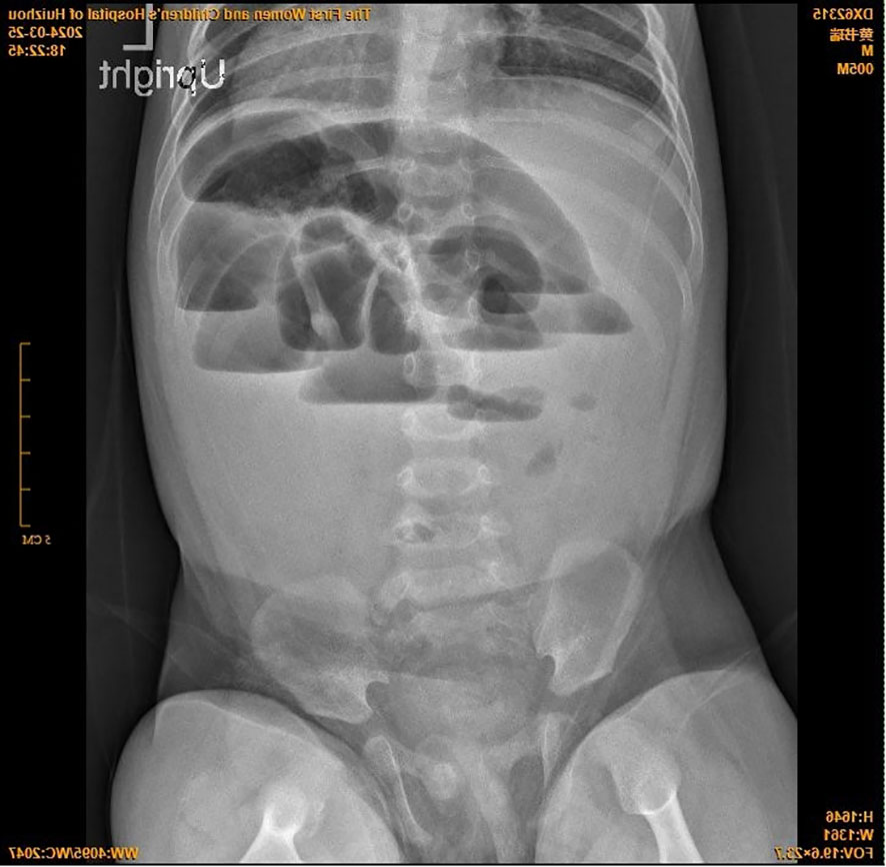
Upright abdominal plain film.
